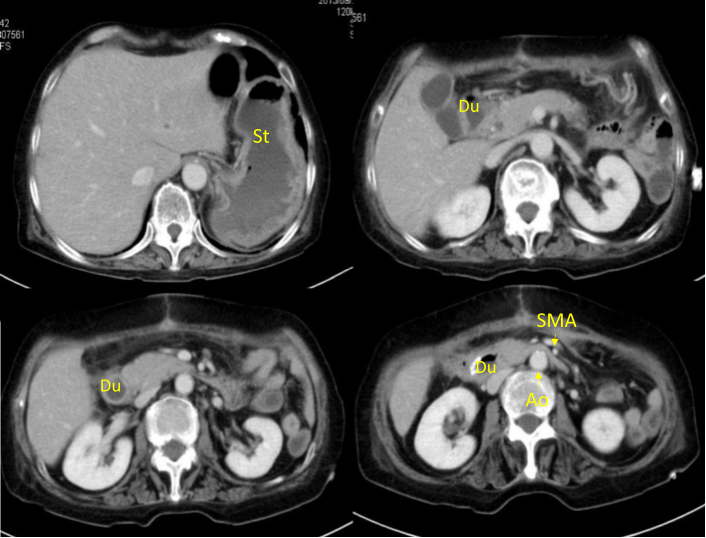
CT after duodenojejunostomy. Fluid collection at the pancreatic stump disappeared early after duodenojejunostomy. Du, duodenum; St, stomach; Ao, aorta; SMA, superior mesenteric artery.
